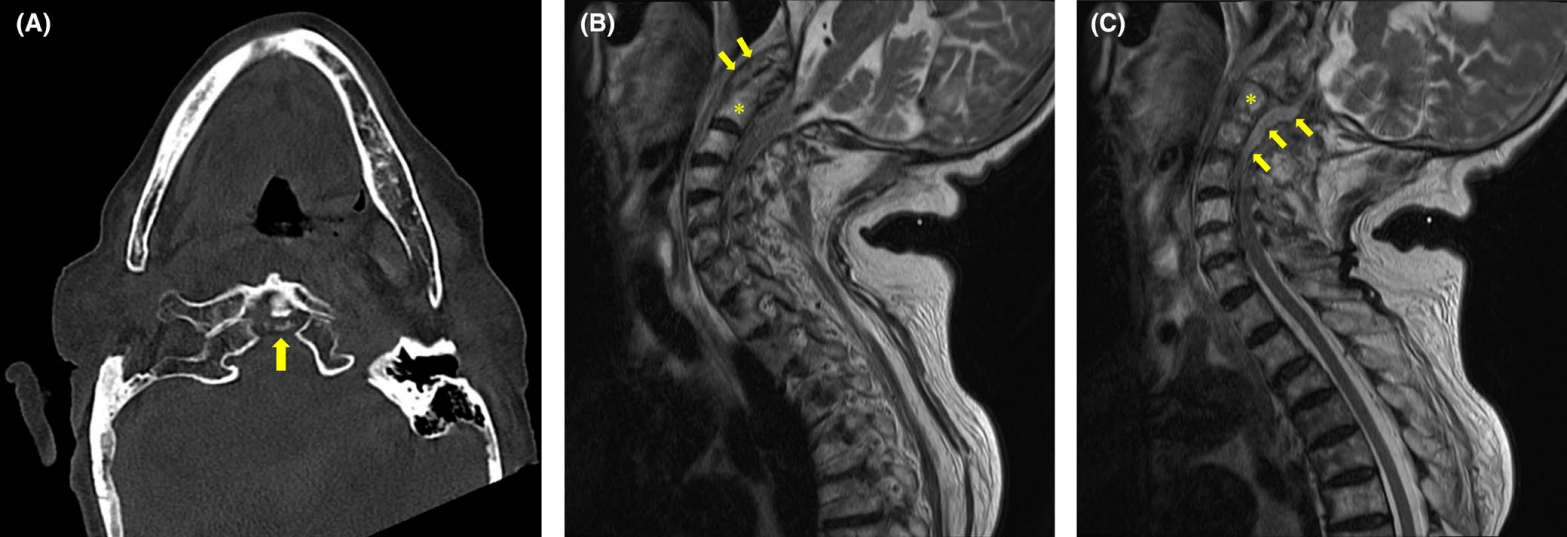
Plain computed tomography scan of the neck showing calcification of the atlantoaxial joint (arrow) (A). Cervical spine T2‐weighted magnetic resonance imaging showing an abscess around the odontoid process (B) and a spinal epidural abscess (C). The asterisk indicates the C2 vertebra.
